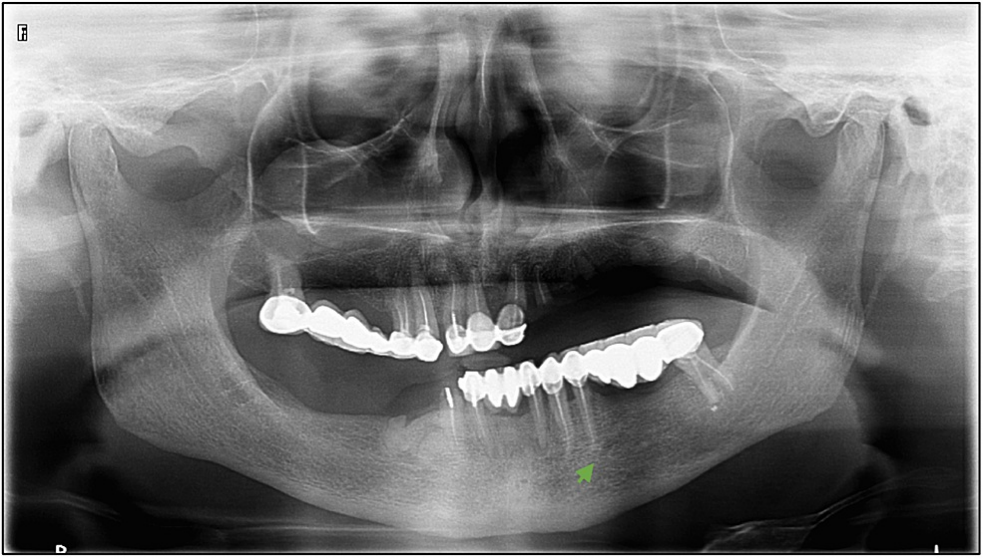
Anterior loop detection in a panoramic radiograph, anterior loop detected on left side (green arrow)
